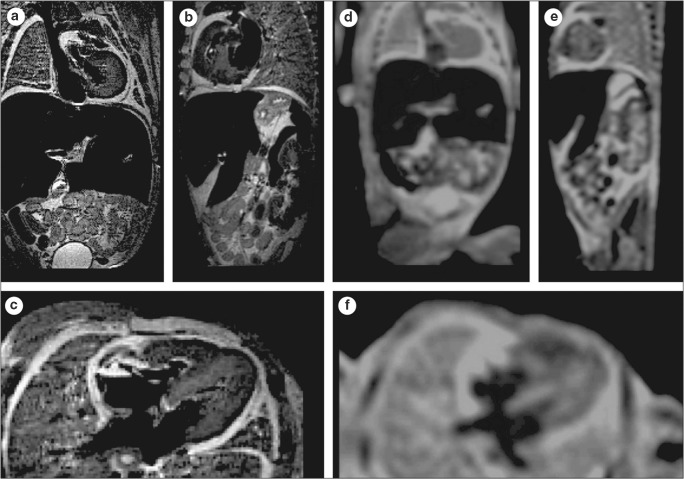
UHF-MRI and low-field MRI images of the same fetus. a Coronal, (b) sagittal, and (c) axial images obtained with three-dimensional T2-weighted MRI at 9.4 T. d Coronal, (e) sagittal, and (f) axial images obtained with three-dimensional T2-weighted MRI at 1.5 T. Figure adapted from *Post-mortem examination of human fetuses: a comparison of whole-body high-field MRI at 9.4 T with conventional MRI and invasive autopsy (Thayyil et al. 2019)* [14] with permission form Elsevier
